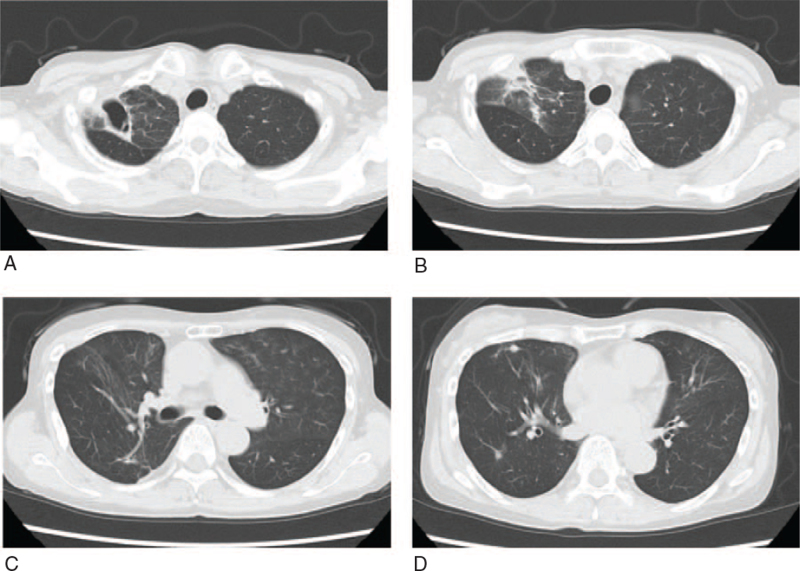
Chest computed tomography findings 3 months after diagnosis. A, B: Consolidation surrounding the cavity in the right upper lobe spread. C, D: Consolidation and patchy opacities in the bilateral lower lobes improved.
